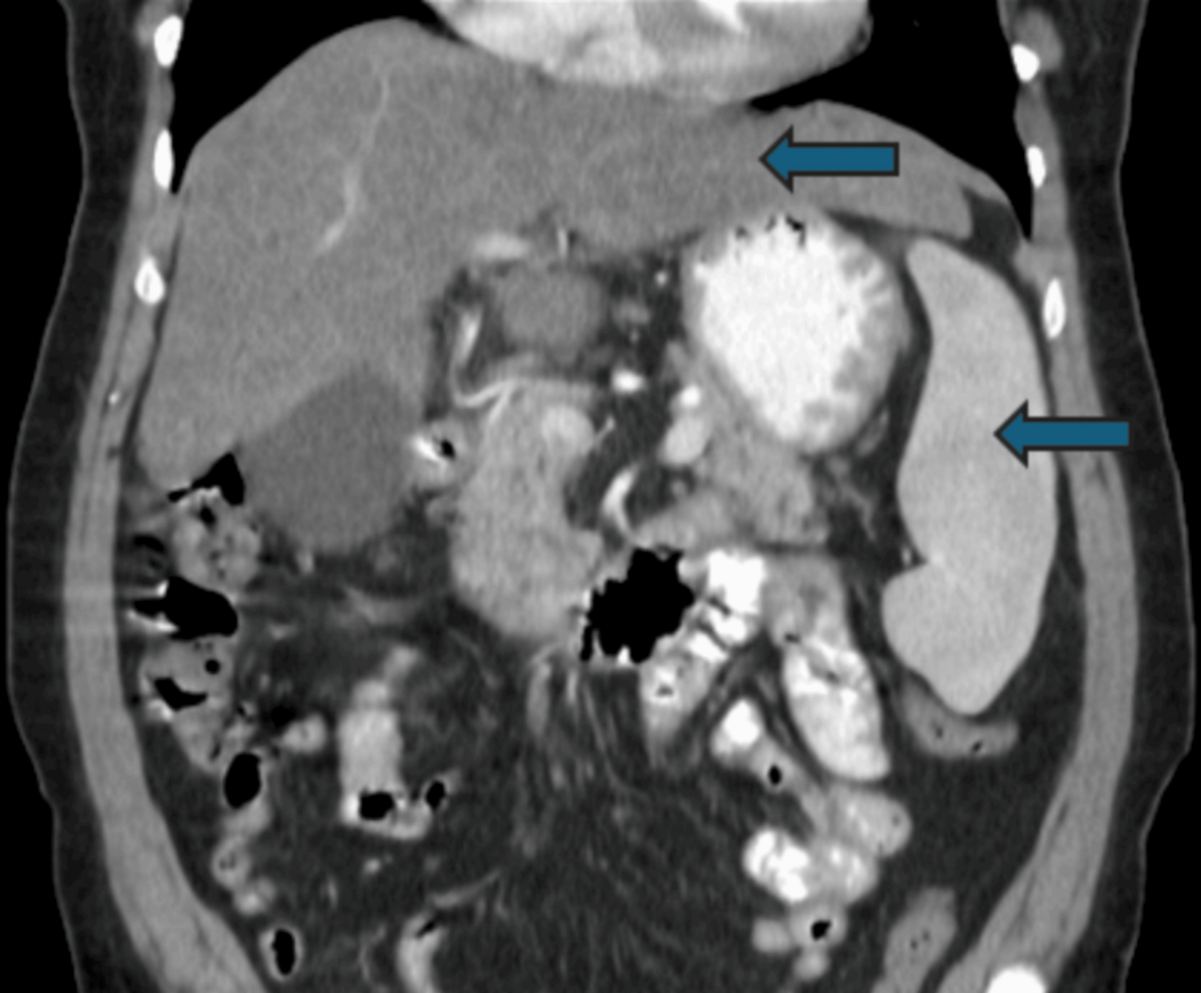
CT scan revealing hepatosplenomegaly (blue arrows)
